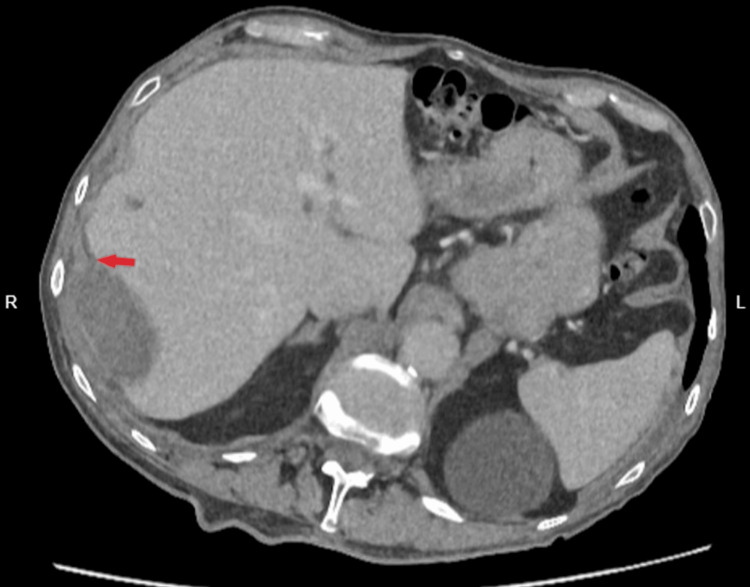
Contrast-enhanced CT abdomen and pelvis with contrast on Day 107 (postdischarge) showing regressing hematoma A small area of residual low attenuation is seen within the liver at the site of the previous drain. The remaining abscess cavities have completely resolved. The hematoma continues to shrink CT: computed tomography
